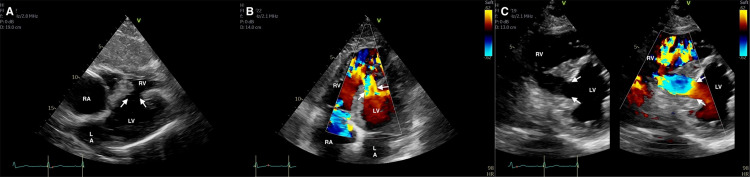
Transthoracic echocardiography. (A) Subcostal view of the ventricular septal rupture in 2D (arrows). (B) Apical four-chamber view of the ventricular septal rupture with left-to-right shunting on color doppler (arrows). (C) 2D with color flow comparison in the parasternal short-axis view demonstrating a large VSD with a significant left-to-right shunt (arrows). LA, left atrium; LV, left ventricle; RA, right atrium; RV, right ventricle.
